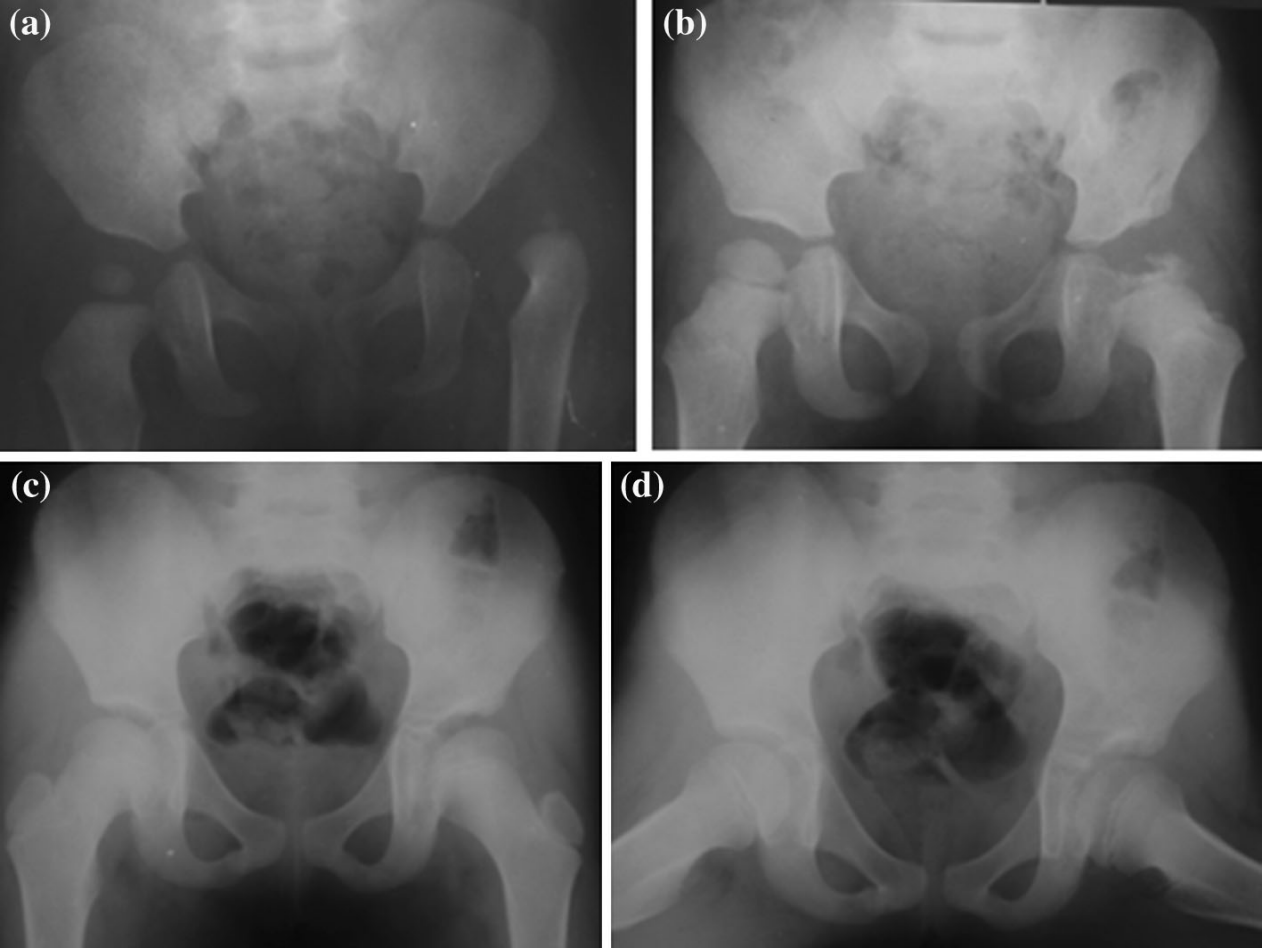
a Pelvis X-ray of 18-month-old girl, b 2-year post-operative pelvis X-ray shows type-1 avascular necrosis (AVN), c, d anteroposterior pelvis X-rag (c) and frog-leg X-ray (d) at 9 years of age shows no sign or sequel of AVN
